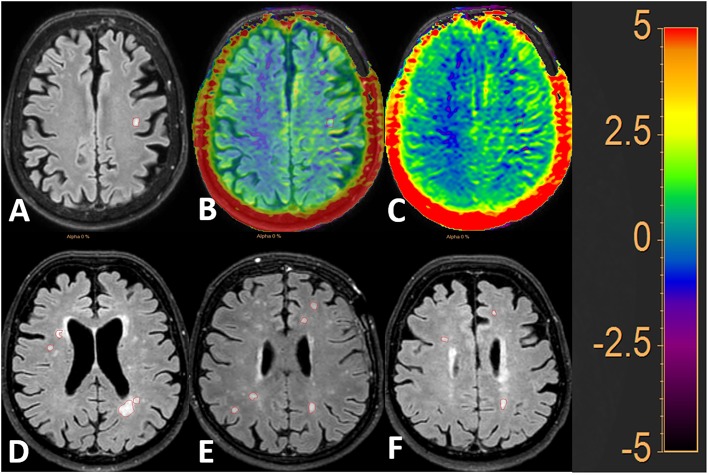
FLAIR image with 50 and 100% APTw image overlay. White matter hyperintensities of presumed vascular origin (WMH) are outlined in red. The image to the left shows the lesions on FLAIR (A), the middle image shows the FLAIR image with 50% APTw overlay (B) and the image to the right shows the FLAIR image with 100% APTw overlay (C). Additional examples of the lesion selection are given in (D–F).
